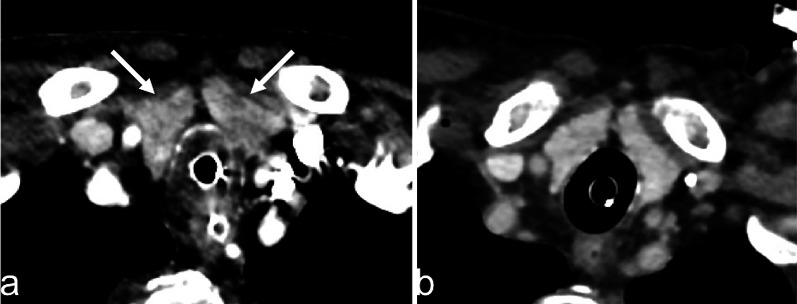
Contrast-enhanced CT image in the portal venous phase showing an 89-year-old female with sepsis due to bowel wall perforation, secondary to colon cancer (qSOFA 3). An inhomogeneous enhancement of the thyroid gland was observed (a, arrow), in contrast with the previously homogeneous enhancement of the gland 4 days earlier (b)
